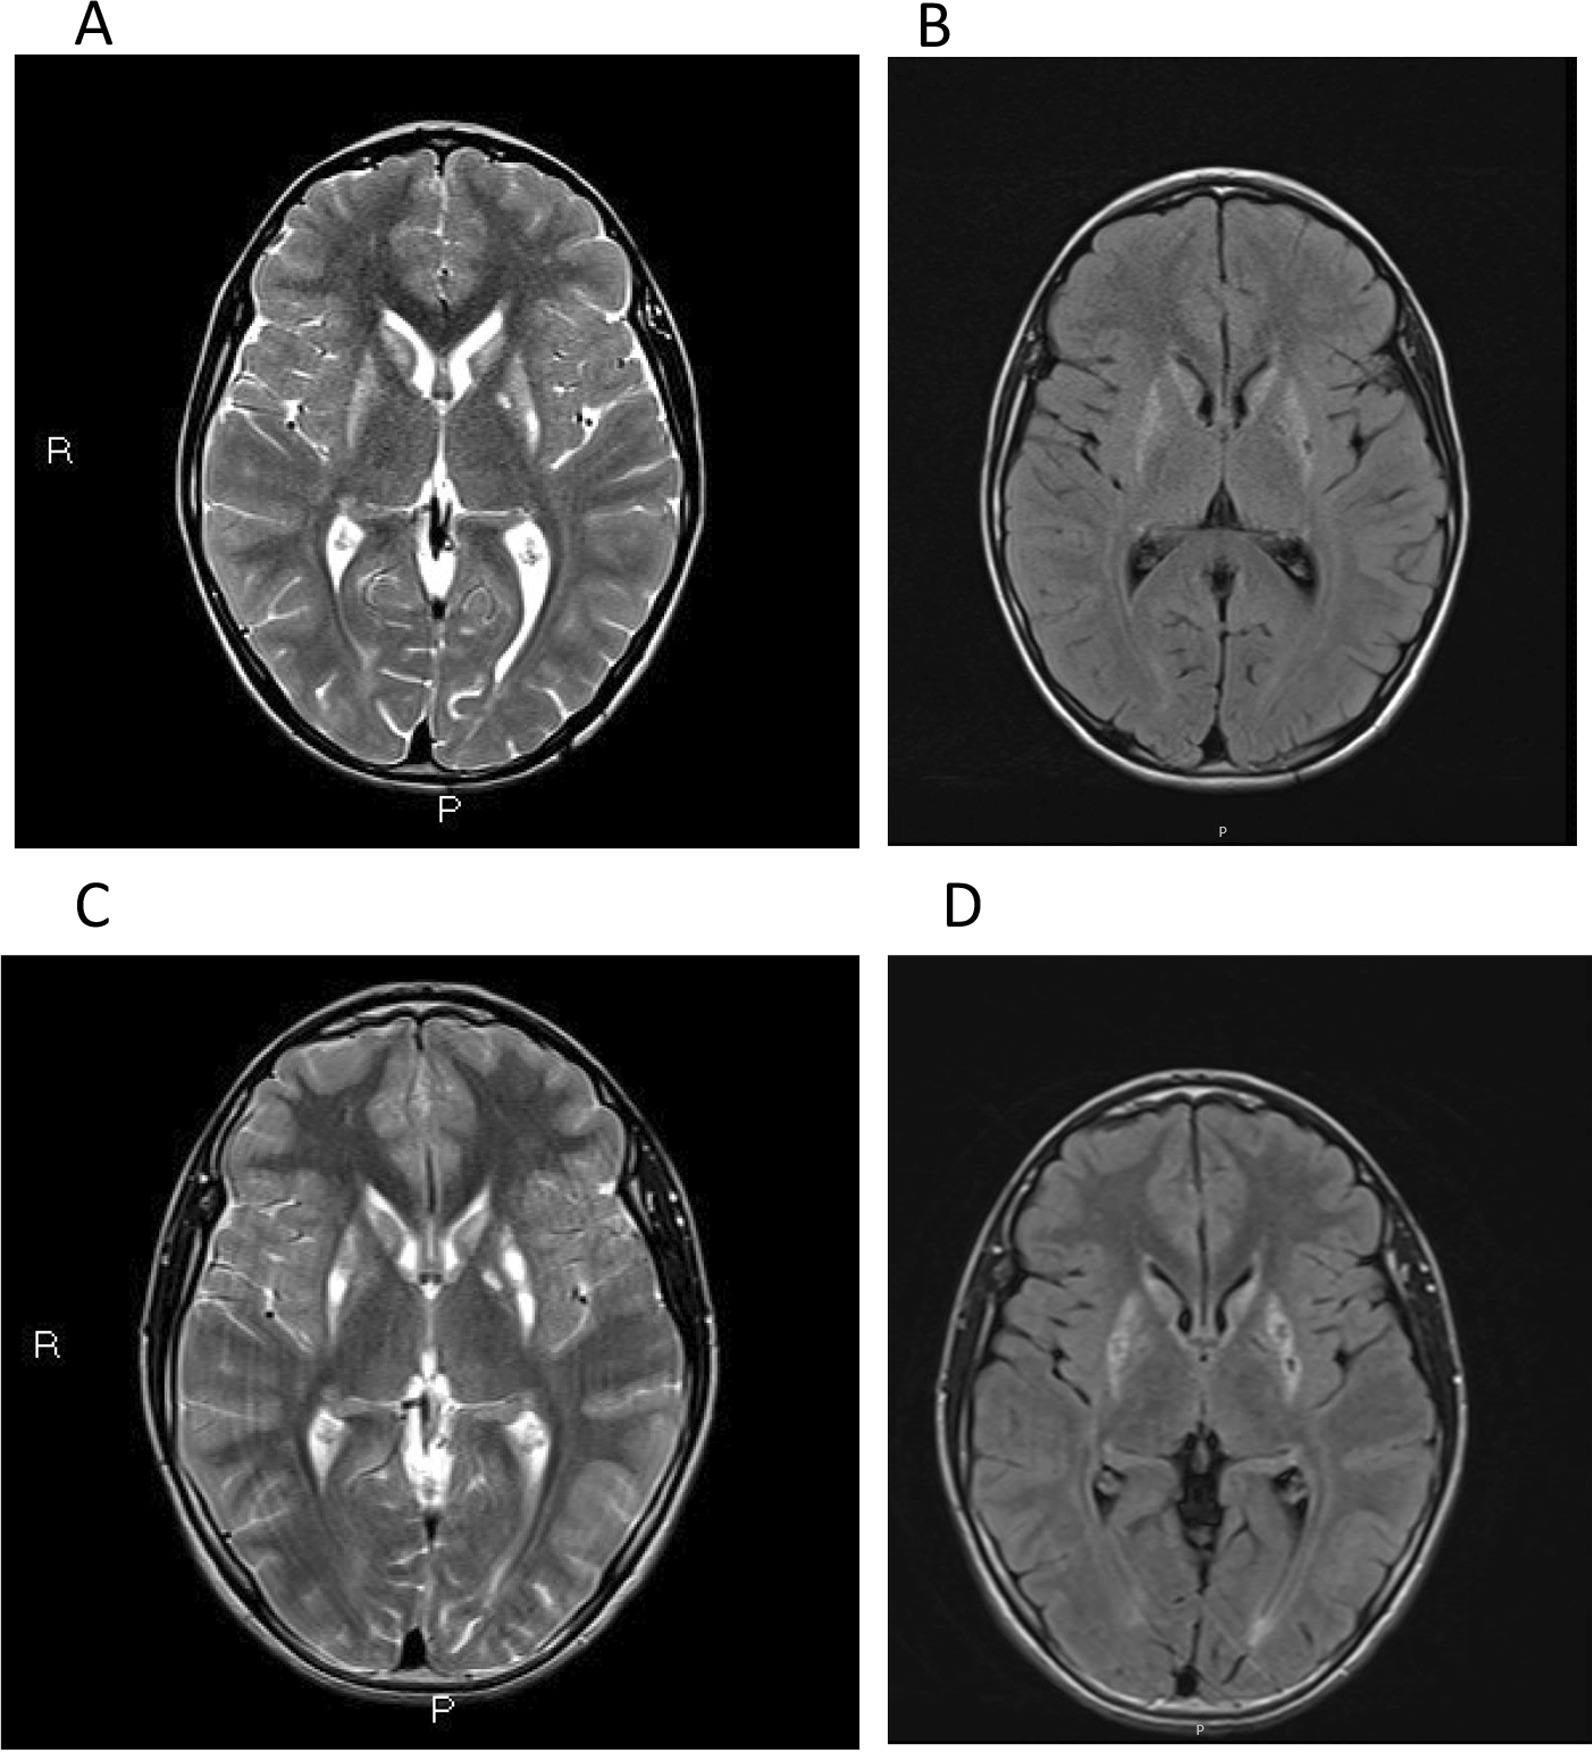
The axial T2 sequence in cerebral magnetic resonance at diagnosis (Panel A) and after 3 years of dietary intervention (Panel C) and the corresponding fluid-attenuated inversion recovery (FLAIR) images (Panel B, D)
